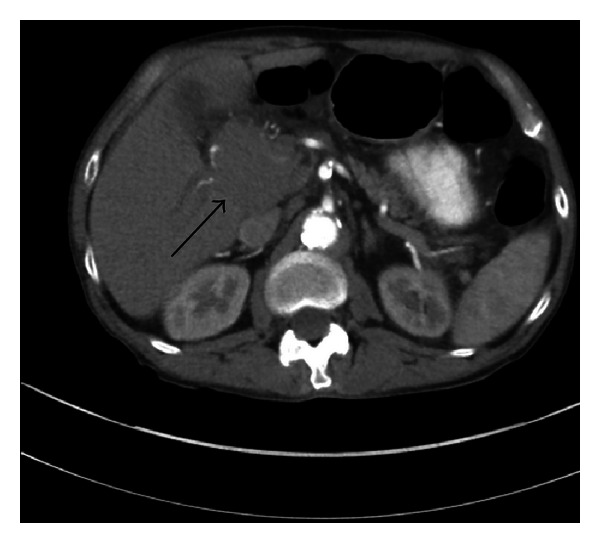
Case 1—MRI abdomen pelvis revealed 5.5 × 5.4 × 4.9 cm mass and biliary ductal dilatation from extrinsic compression (black arrow).
